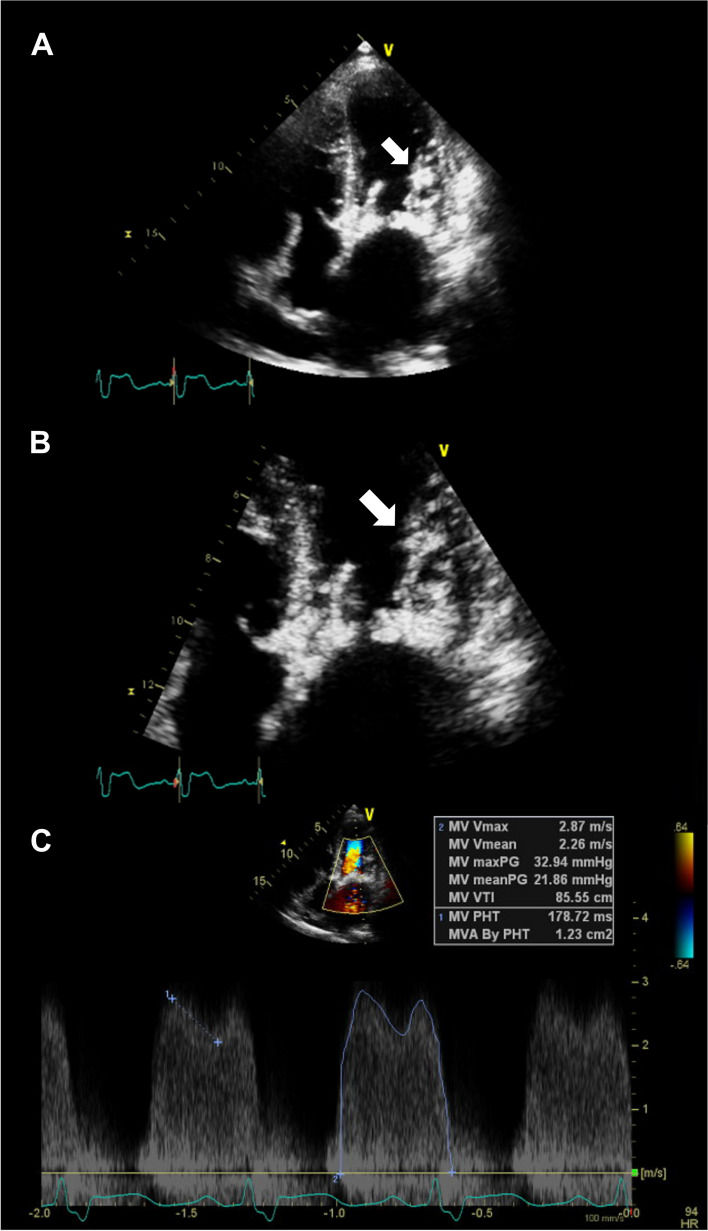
Apical view on transthoracic echocardiogram. A Thickened MV with subvalvular mass-like materials (white arrow). B Zoomed view of the MV with subvalvular mass-like materials (white arrow). C Continuous wave Doppler recordings of mitral inflow with a transvalvular pressure gradient of 21.9 mmHg, suggesting severe mitral stenosis. maxPG: maximum pressure gradient, meanPG: mean pressure gradient, MV: mitral valve, MVA: mitral valve area, PHT: pressure half-time, Vmax: maximum velocity, Vmean: mean velocity, VTI: velocity time integral
